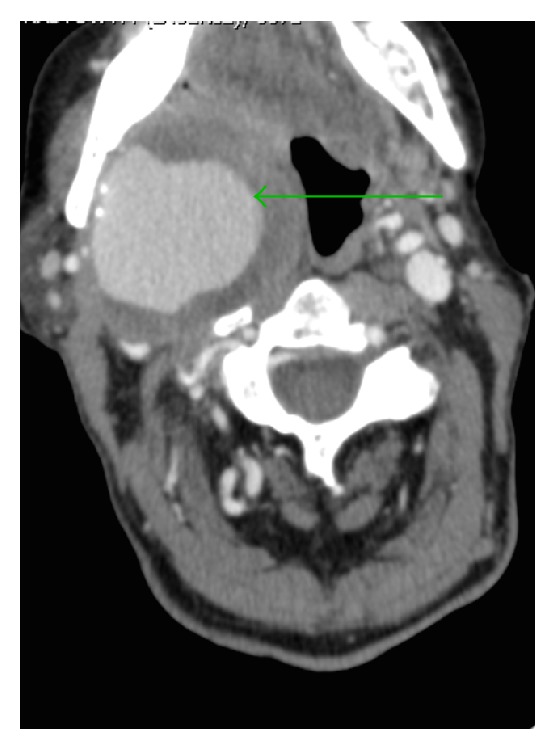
Computed Tomography showing extracranial aneurysm of right internal carotid artery (arrow).
